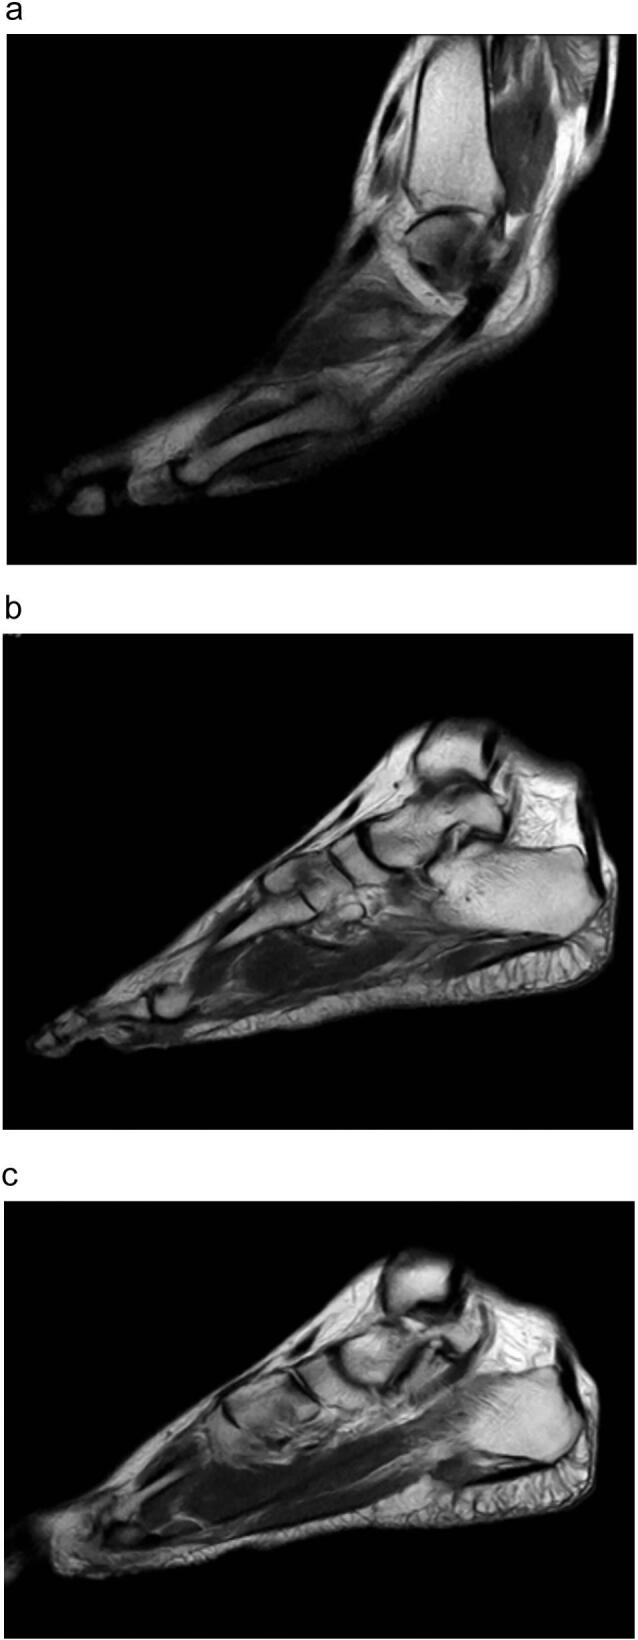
MRI of Morton's Neuroma on the right foot shows thickening of nerve cells on the intermetatarsal space 2nd-3rd.
